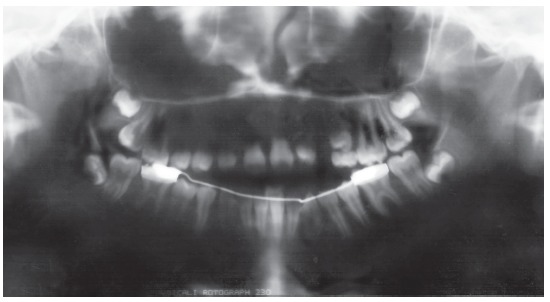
Panoramic radiograph with the lingual arch.
